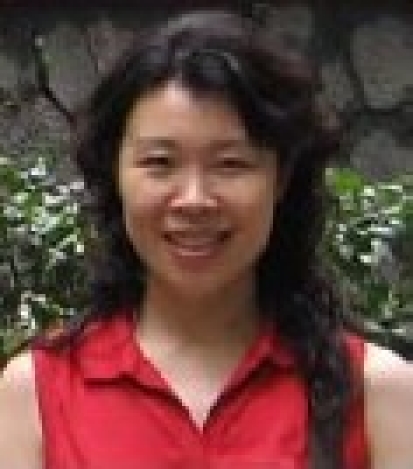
Fang Liu, M.D. Associate Chief Physician, Department of Geriatric Cardiology, Peking University First Hospital. Dr. Liu specializes in clinical cardiology and echocardiogram. Recently, she serves as an editorial member of *Chinese Journal of Multiple Organ Diseases in the Elderly*. She is Echo-Specialist of Peking Physician Association and Director of Bejing Institute of Ultrasound in Medicine
